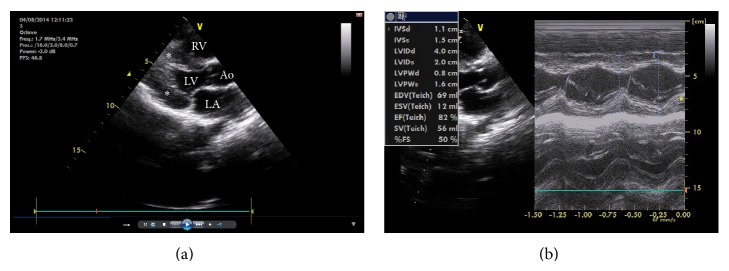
(a) Parasternal long-axis view depicting abnormally small left ventricular end-diastolic cavity, concentric left ventricular hypertrophy (∗), and normal regional wall motion; (b) M-mode tracing across the interventricular septum demonstrating prominent wall hypertrophy. LA: left atrium; LV: left ventricle; RV: right ventricle; Ao: aorta; IVSd: interventricular septum diastolic diameter; IVSs: interventricular systolic diameter; LVIDd: left ventricular end-diastolic diameter; LVISd: left ventricular end systolic diameter; LVPWd: left ventricular posterior wall thicknesses diastolic diameter; LVPWs: left ventricular posterior wall thicknesses systolic diameter; EF: ejection fraction; FS: fractional shortening.
